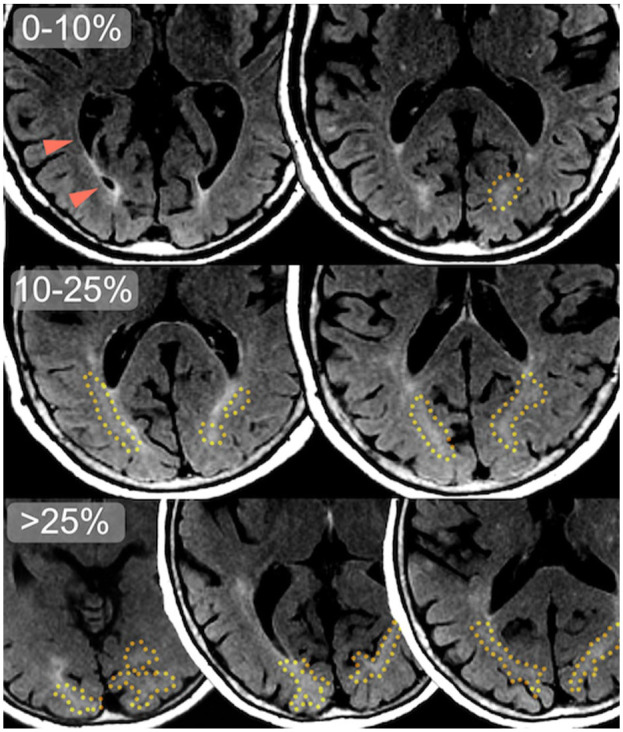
Common locations of occipital DAWM on FLAIR MRI slices. Transversal slices of 1.5 T FLAIR MRI scans of three different older adult participants, with DAWM denoted by a yellow dotted line. Note the difference in apparent DAWM volume between the top row (0%–10% DAWM of total occipital white matter), where only small occipital periventricular WMH and ependymal lining is visible, and the middle row (10%–25% DAWM) and bottom row (>25% DAWM) where larger, bilateral more confluent configurations of occipital DAWM can be seen, extending beyond the slices shown here. The optic radiation is denoted by orange arrowheads: its darker anterior segment is Meyer’s loop curving around the ventricles, while its fanning connections to the cortex taper off with a very subtle, diffuse intensity gradient.
